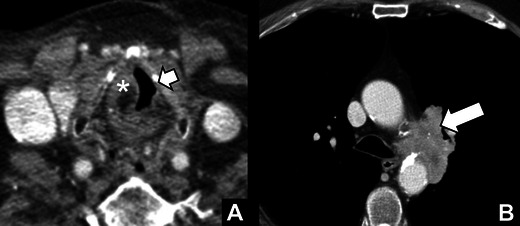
Lung cancer with involvement of the aorto-pulmonary (AP) window.*72 year old male smoker with a history of squamous cell carcinoma of the left ear presenting with functional dysphonia caused by left VCP. a Axial contrast-enhanced CT of the larynx showing complete atrophy of the left true vocal cord (*short arrow*) and hypertrophy of the right true vocal cord (*). b Images at the level of the AP window showed a large supra-hilar mass in the left lung infiltrating the AP-window (*arrow*), thereby explaining left VCP in this patient. Biopsy revealed a non-small cell lung carcinoma
